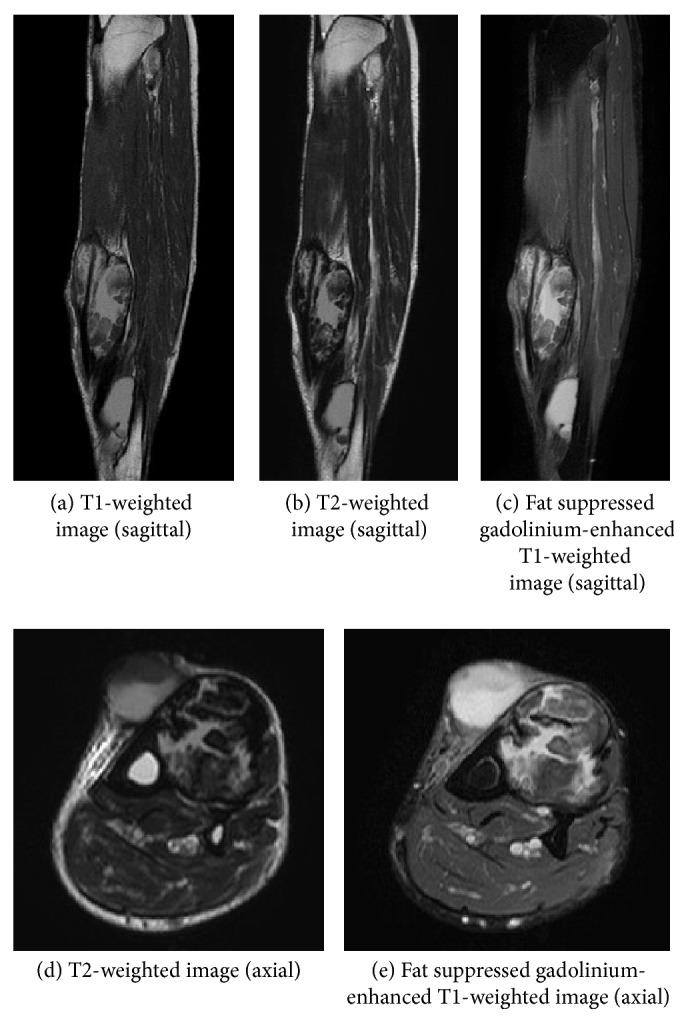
MRI revealed that the mass exhibited heterogeneous signals on T1- and T2-weighted images and the cystic lesions exhibited homogeneous signals on T1- and T2-weighted images (a, b, and d). Fat suppressed gadolinium-enhanced T1-weighted images revealed slight heterogeneous enhancement within the affected muscle with focal hypointense nonenhancing areas (c and e).
